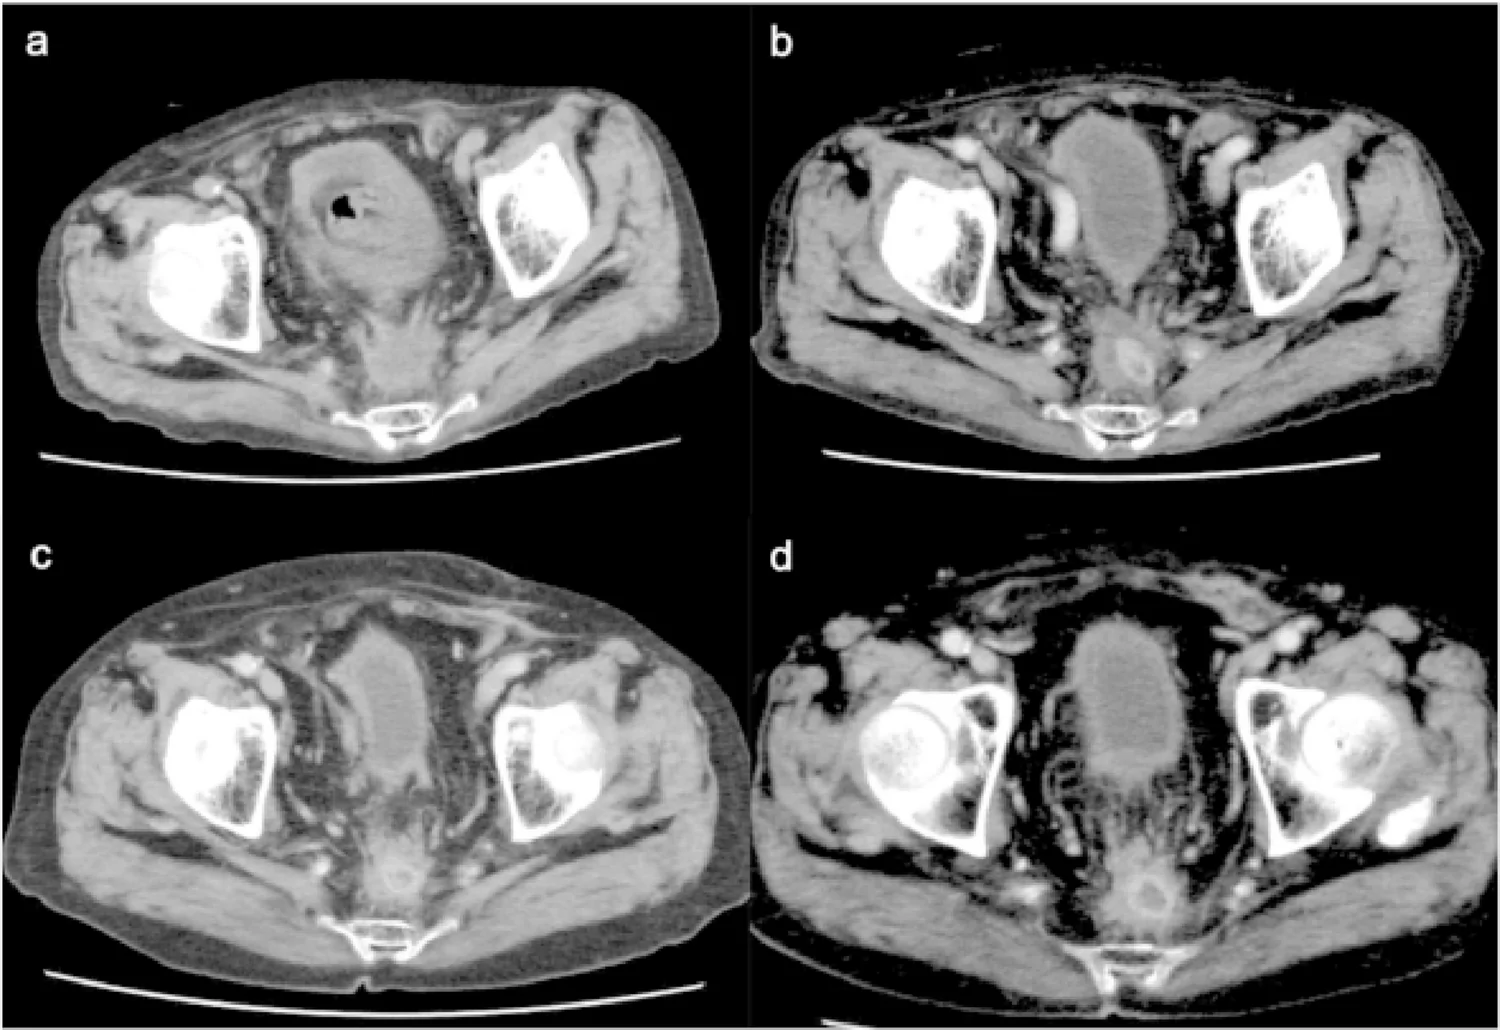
Chronological changes in the presacral soft-tissue lesion with an enhancing nodule after surgery. Plain CT obtained 3 months after surgery is shown in panel a. Contrast-enhanced CT obtained 12, 24, and 27 months after surgery is shown in panels b, c, and d, respectively
